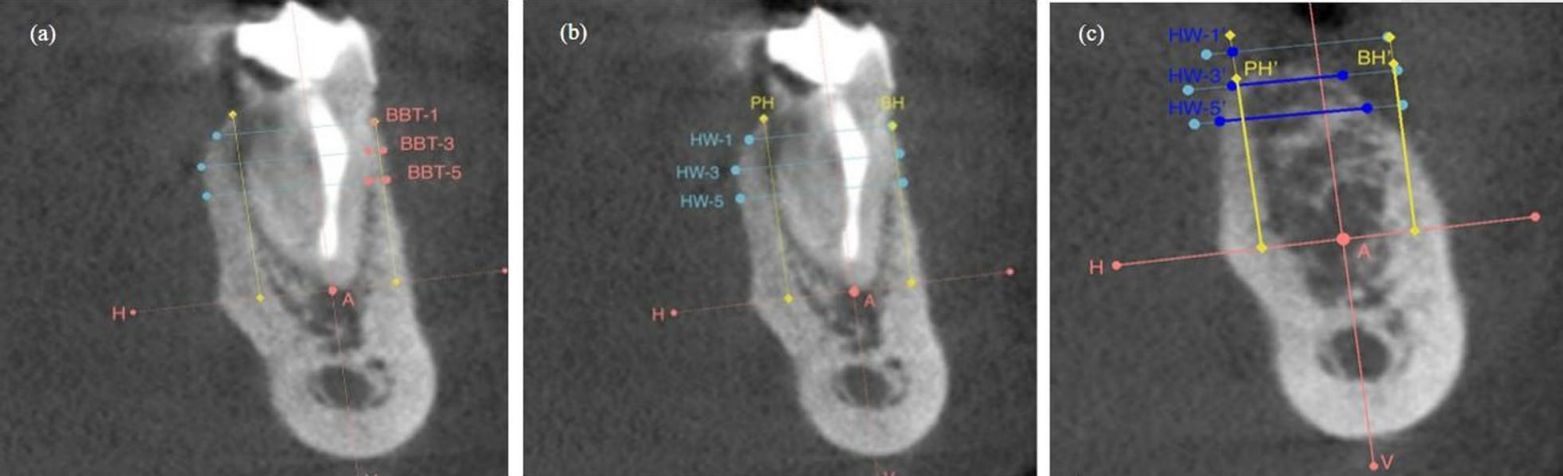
Linear measurements on CBCT images of the case treated following the spontaneous healing protocol. a) Preoperatively the horizontal analysis was performed at 1, 3 and 5 mm (HW-1, HW-3, HW-5) and the vertical analysis was performed on the buccal and palatal side (BH, PH); b) preoperatively the buccal bone thickness was measured at 1, 3 and 5 mm (BBT-1, BBT-3, BBT-5); c) postoperatively the horizontal analysis was performed at 1, 3 and 5 mm (HW-1, HW-3, HW-5) and the vertical analysis was performed on the buccal and palatal side (BH, PH)
